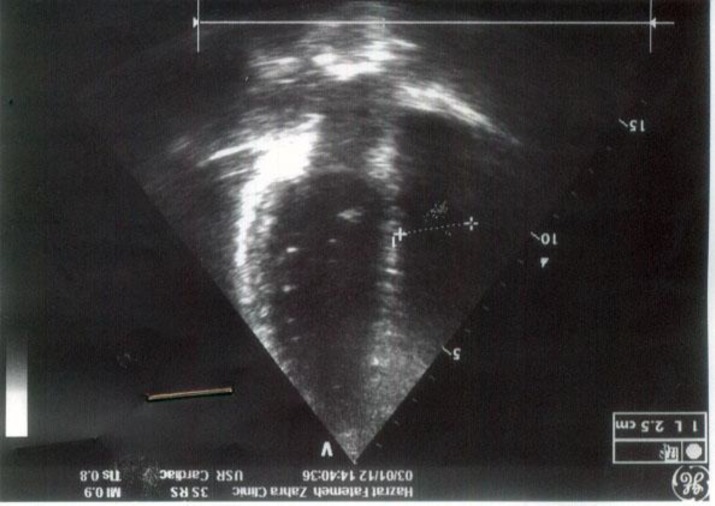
Echocardiography: Normal mitral valve without regurgitation, and no occurrence of tumor
